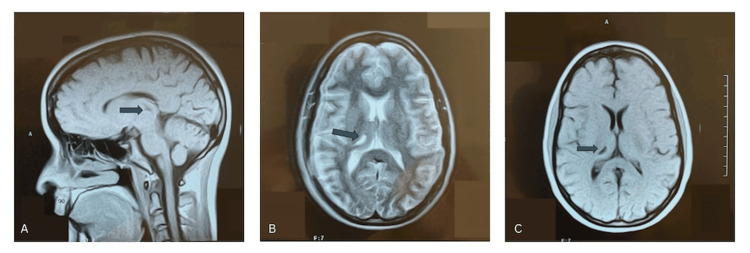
1997 MRI Brain Imaging (A) Coronal T1-weighted sequence; (B) Axial T2-weighted sequence; (C) Axial T1-weighted sequence.
